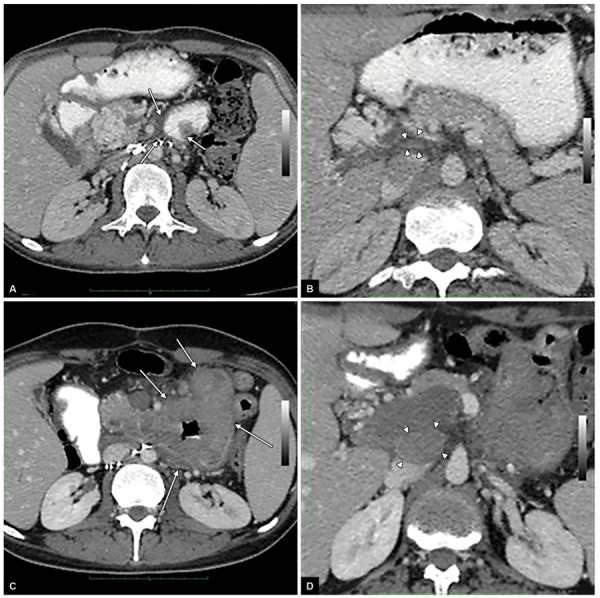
Abdominal CT applied before treatment showed metastatic duodenal mass on the right (C and D arrows). After treatment with a chemotherapy regimen composed of cisplatin, paclitaxel, and ifosfamide, a follow-up CT examinations revealed that there was a prominent regression in the metastatic mass and the enlarged lymph nodes seen on the left (A and B arrows)
